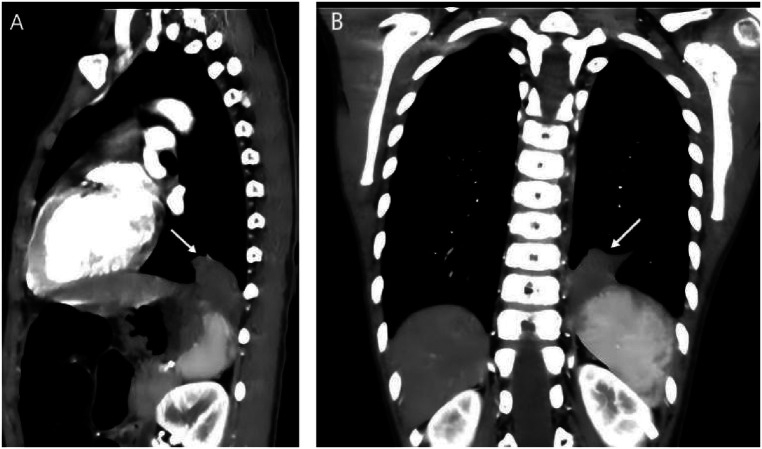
Contrast-enhanced chest CT. (A) A soft-tissue mass (arrow) is noted with well-defined margins. (B) A soft-tissue mass (arrow) is shown with no definite arterial feeding vessel identified.
